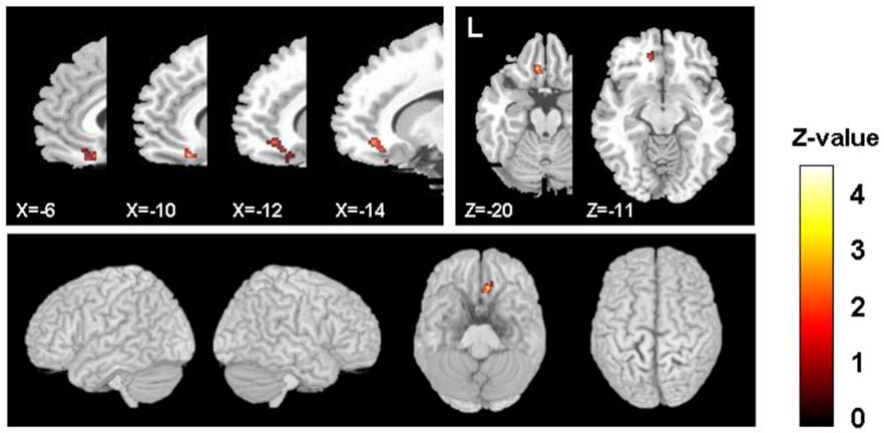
Sagittal and transverse sections of the statistical parametric map of the changes in [^11^C]FLB 457 BP after left DLPFC stimulation overlaid upon the standard MRI.
